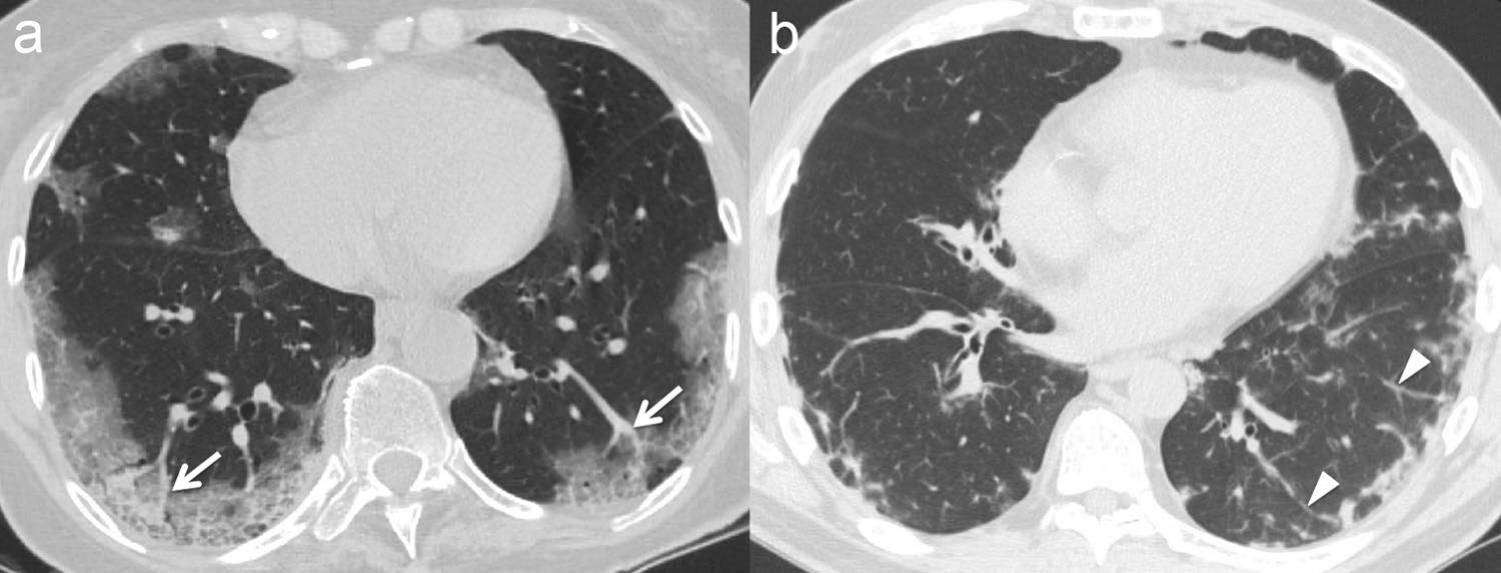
a Patient with COVID-19 pneumonia. The CT scan shows a crazy-paving pattern with typical predominant subpleural and bilateral distribution at the lung bases; note the presence of "enlarging vessel" sign within the areas of increased lung density (arrows). b Patient with H1N1 influenza-associated pneumonia. The CT scan shows multifocal small areas of consolidation and perilobular opacities with bilateral subpleural distribution at the lung bases, associated with some GG areas in the left lower lobe; note the normal caliber of pulmonary vessels in the proximity of the pulmonary abnormalities (arrowheads)
